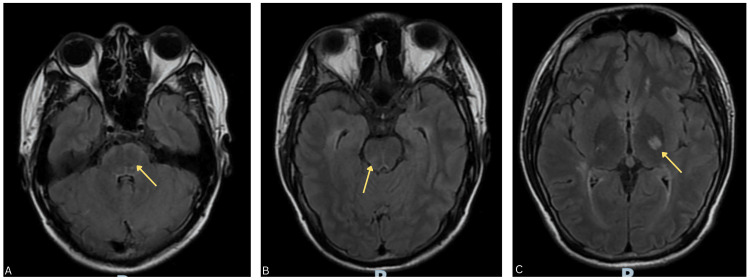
Characteristic brain MRI findings in a 32-year-old male with multiple sclerosis Axial fluid-attenuated inversion recovery (FLAIR) sequences demonstrating typical focal hyperintense demyelinating plaques (indicated by yellow arrows). A: hyperintense lesion located in the left pons; B: lesion in the right cerebral peduncle (midbrain); and C: lesion involving the left thalamus and adjacent deep white matter.
